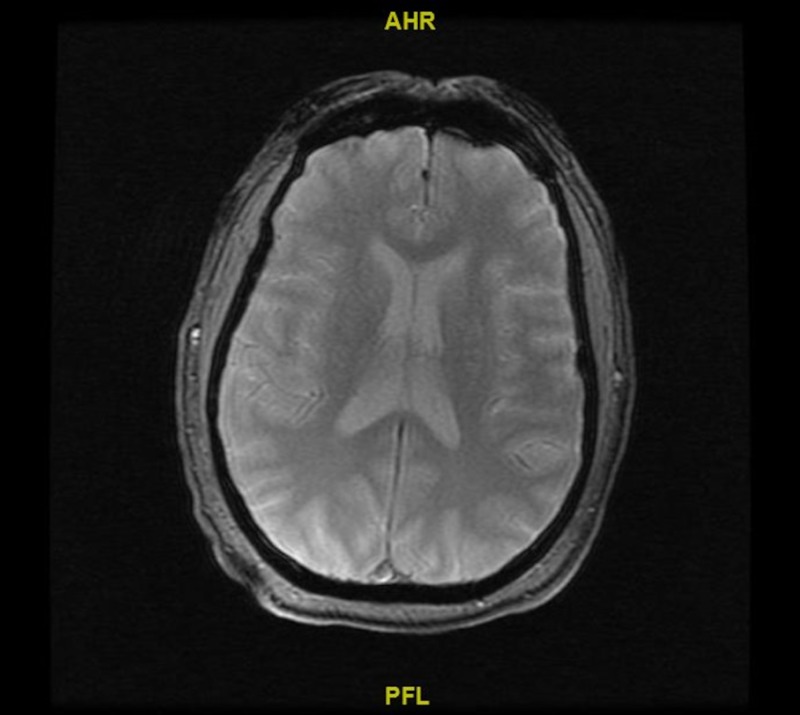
Magnetic resonance imaging (MRI) of the brain No acute intracranial pathology can be seen in the scan.
